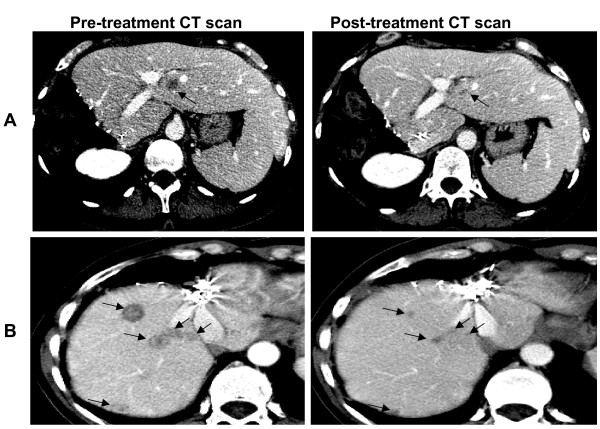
Objective partial responses to cetuximab-based treatment in patients with *KRAS- *mutated tumors. (A) Pre- and post-cetuximab CT scan showing partial tumor response allowing surgical resection to be performed in patient 6. (B) Pre- and post-cetuximab CT scan demonstrating a major tumor response to cetuximab-based treatment in patient 7.
